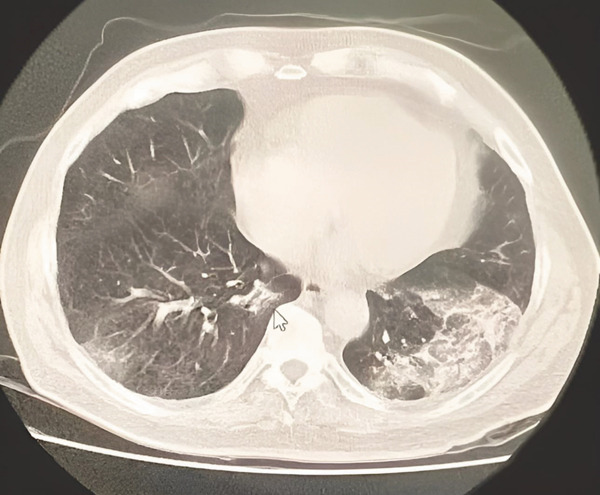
Noncontrast CT scan from the day of metamizole exposure.
